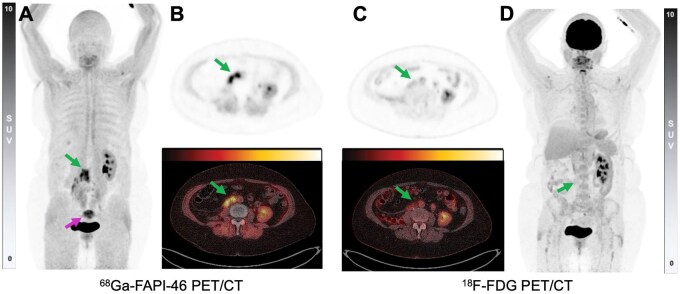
A 63-year-old woman with a local recurrence of a pleomorphic liposarcoma in the right psoas muscle. (A and B) ^68^Ga-FAPI-46 PET/CT shows the local recurrence (green arrow) and physiological intrauterine uptake (pink arrow). (C and D) ^18^F-FDG PET/CT shows the local recurrence (green arrow) as almost non-avid. Maximum intensity projection PET (A and D), axial PET (B and C, top), and axial fusion PET/CT (B and C, bottom). Intrauterine uptake on FAPI PET/CT is a pitfall and tends to decrease after the menopause, although it can be very intense in women of childbearing age.^13^ PET acquisitions were performed on the same day, and the study was performed as part of a basket trial at Universitätsklinikum Essen, Klinik für Nuklearmedizin from the EudraCT 2021-000148-23 trial.
